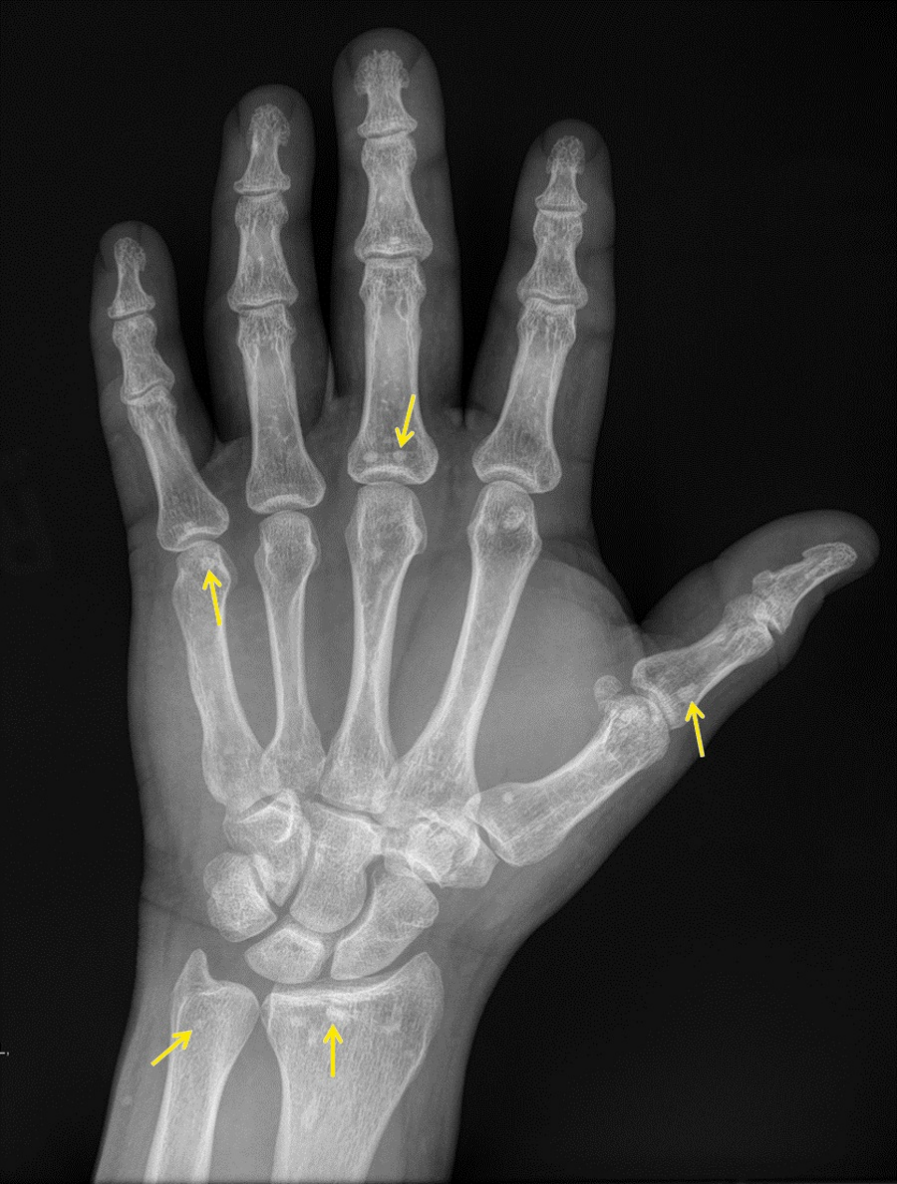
Anteroposterior Radiograph of the Left Hand. Multiple small, non-aggressive, periarticular sclerotic foci (yellow arrows) are visualized at the distal radius, distal ulna, first carpometacarpal joint, first through fifth metacarpal phalangeal joints, second and third proximal interphalangeal joints, and fifth distal interphalangeal joint.
